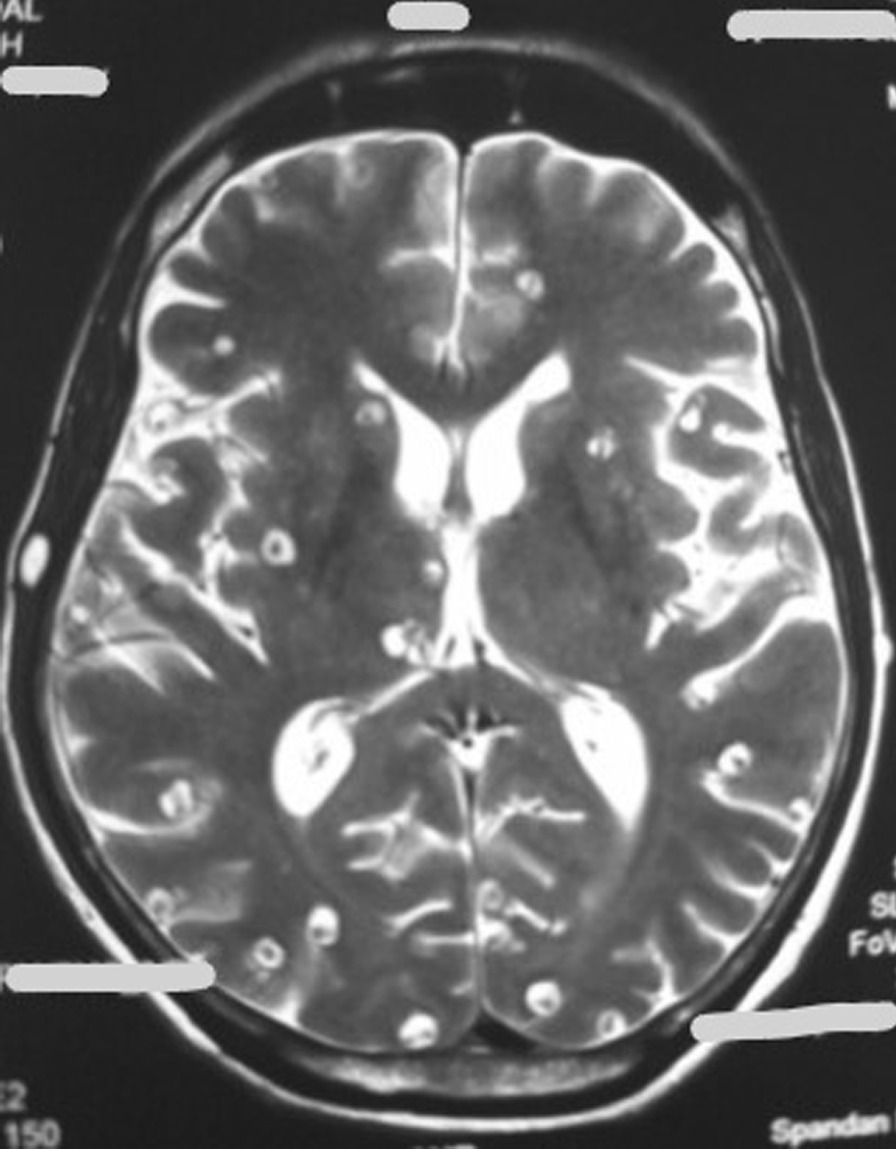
Magnetic Resonance Imaging of brain (T2 sequence) showing multiple cysts with scolex contained therein
